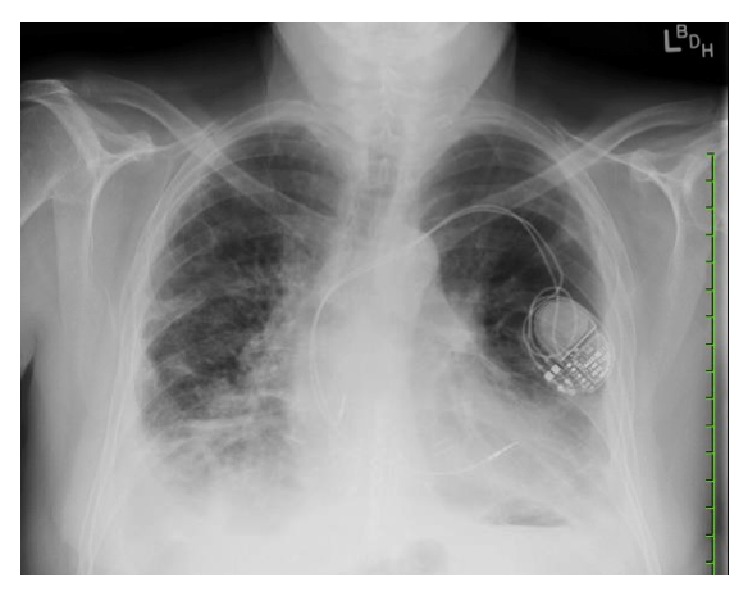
Chest radiography after two weeks of antifungal therapy showing right lower lobe opacity.
